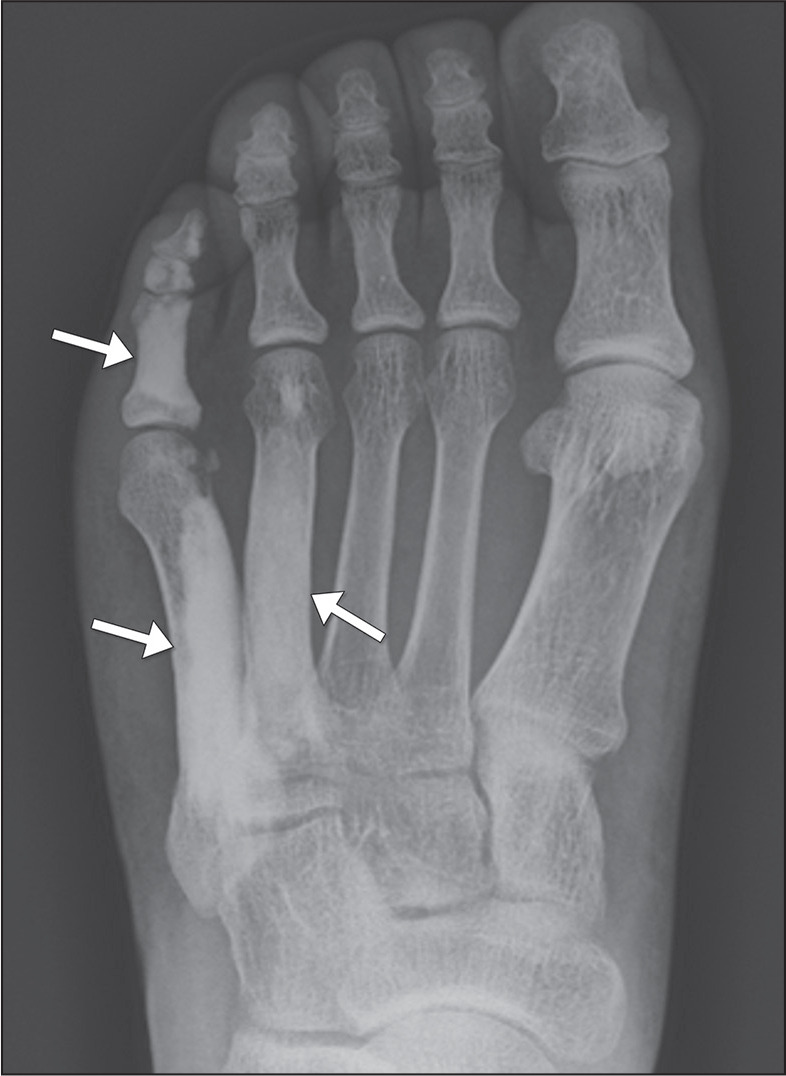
Anteroposterior X-ray of the left foot of a 39-year-old patient
